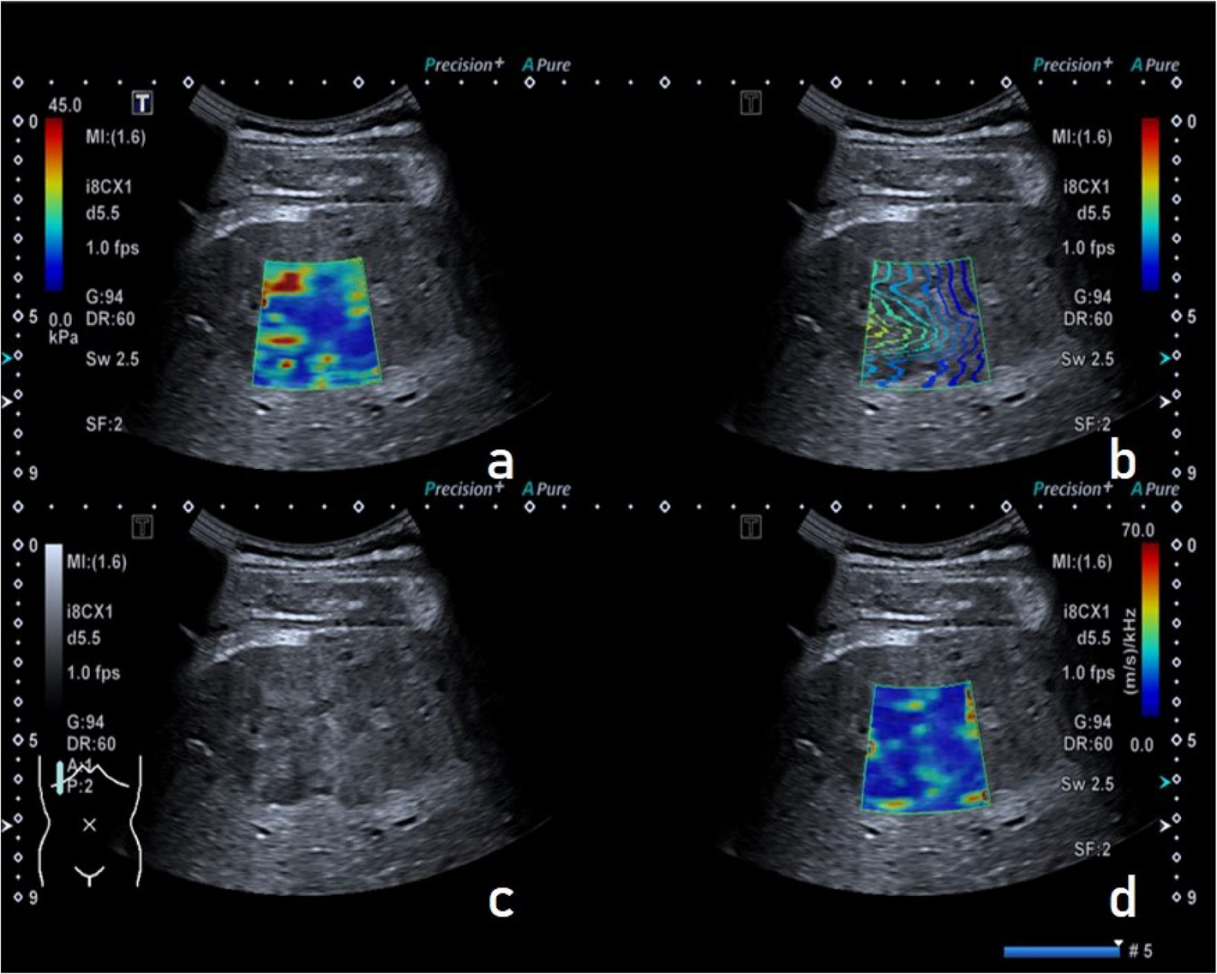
Multi-modal colour ultrasound image. a. Elastography. b. Propagation map, which reflects the image quality. c. Gray-scale ultrasound. d. Viscosity modality
